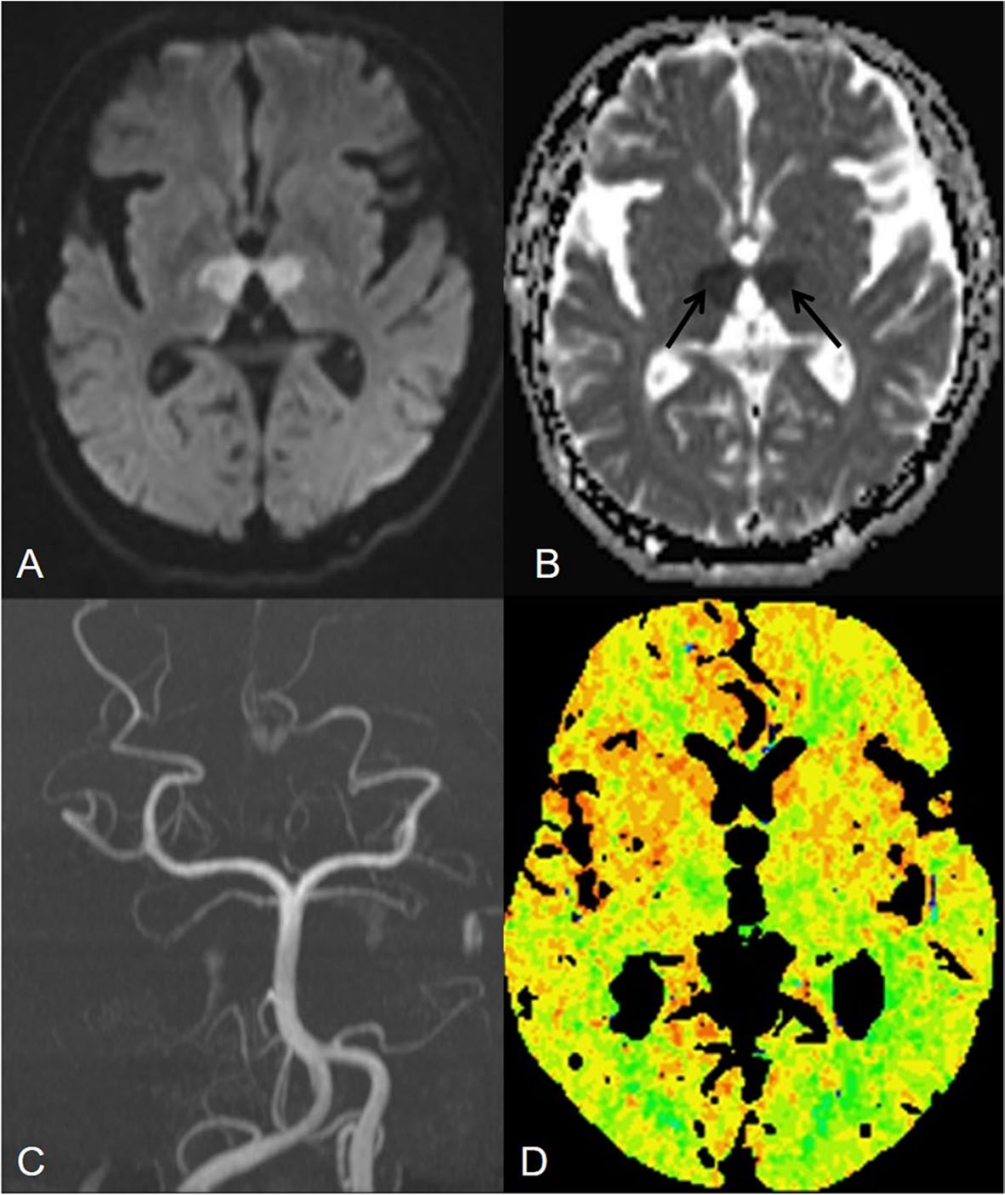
Acute symmetric infarctions in the bilateral paramedian thalamus on MRA and CT perfusion. Case 23. Axial DWI and ADC map (A and B) show acute symmetric infarctions in the bilateral paramedian thalamus (black arrows). MRA demonstrate no occlusion or stenosis in the posterior circulation (C). Hyperacute CT perfusion imaging with white arrows identifying areas of decreased mean blood flow (D)
